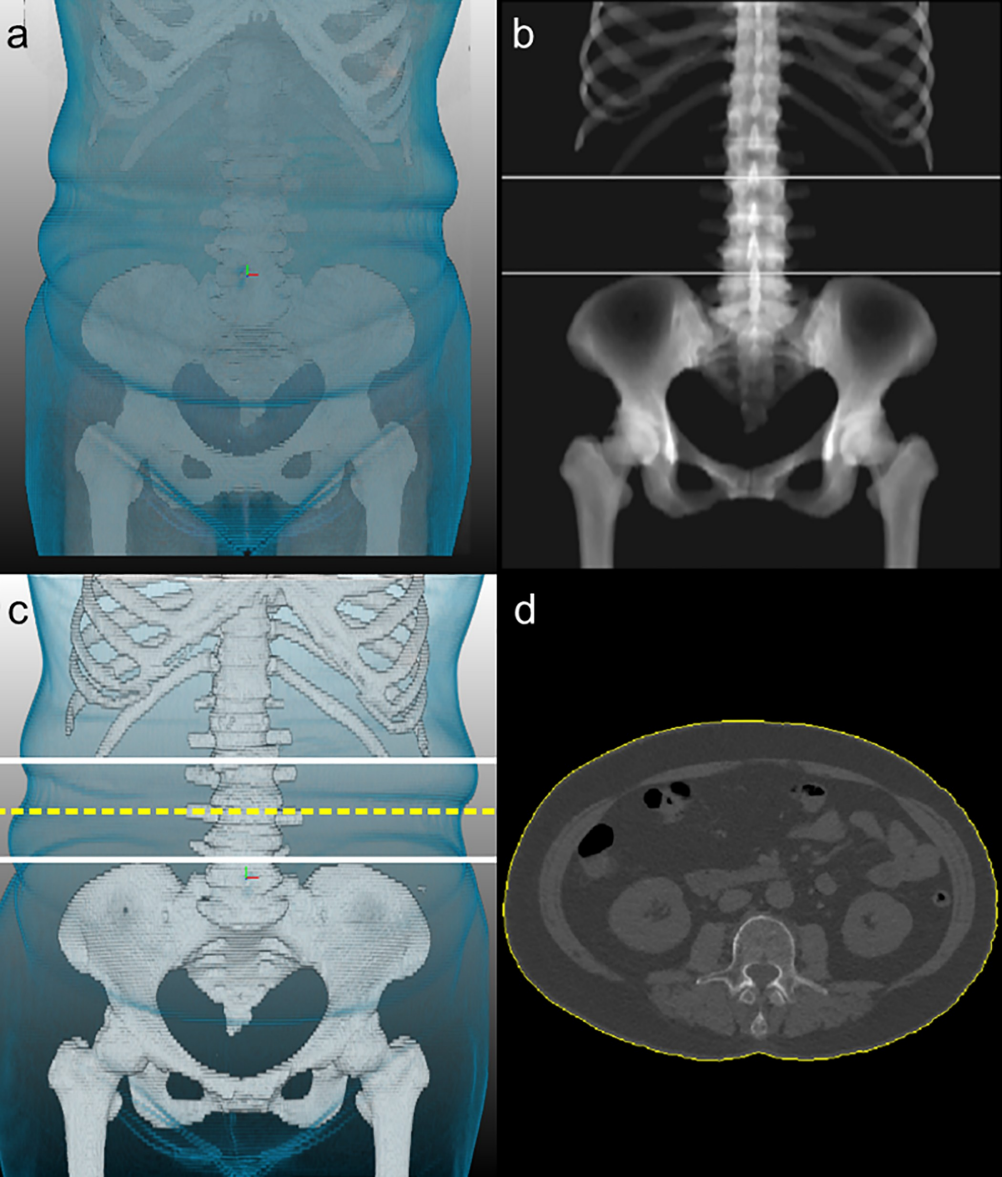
Example of a CT measurement of waist circumference using the fully automated body segmentation algorithm. (a) 3D display of skin and bone, (b) identification of the z-axis level of the lowest rib margin and the highest iliac crest margin, (c) determination of the midpoint z-axis level (dashed line) between the lowest rib margin and the highest iliac crest margin, (d) extracted body perimeter (yellow line) in the selected axial image based on 3D skin segmentation.
